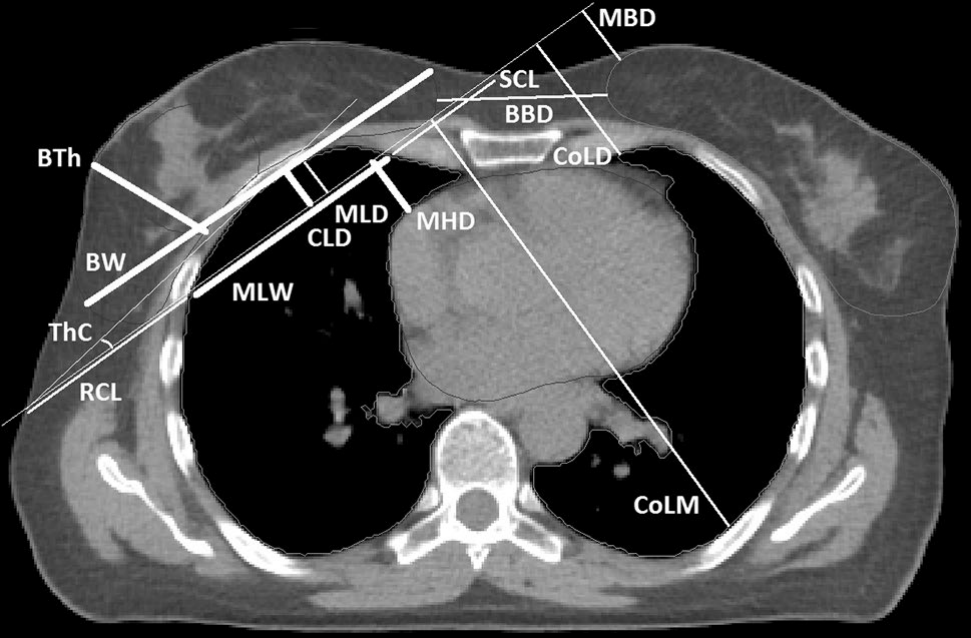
Anatomic measures scored from patients’ CT images. Cranio-caudal measures are not shown.
